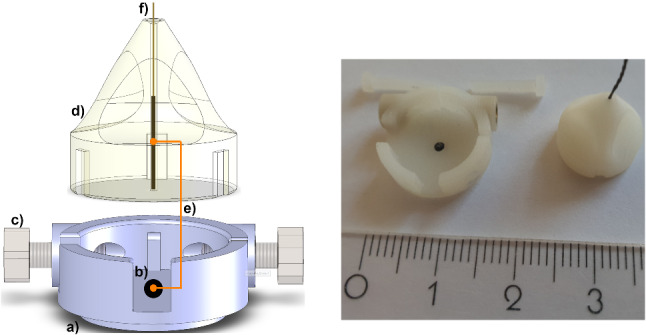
Left: (a) Main part of SH. (b) A X-Spot CT marker placed in the main part concentrically. (c) Two M2 screws are applied from both directions to establish a rigid setup once an EM-SH is placed in the main SH. (d) The EM-SH holds the tracking sensor perpendicular to CT marker and plugged into the main part, so that the offset (e) between sensor and CT marker center is determined. (f) 5D tracking sensor is fixed in the EM-SH concentrically. Right: SH with an EM-SH and tracking sensor shown in cm. The parts (a) and (b) are intended to use for off-line prediction after image acquisition and all together in intraoperative real-time prediction. The SHs are distributed homogeneously on the surface with $$\approx 3~\hbox {cm}$$ distance
